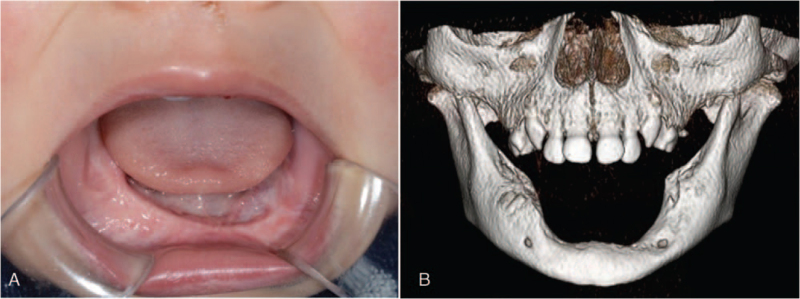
Postoperative state. (A) Intraoral photography. (B) 3D reconstruction of CT images 1 year after surgery revealed no evidence of recurrent tumor.
